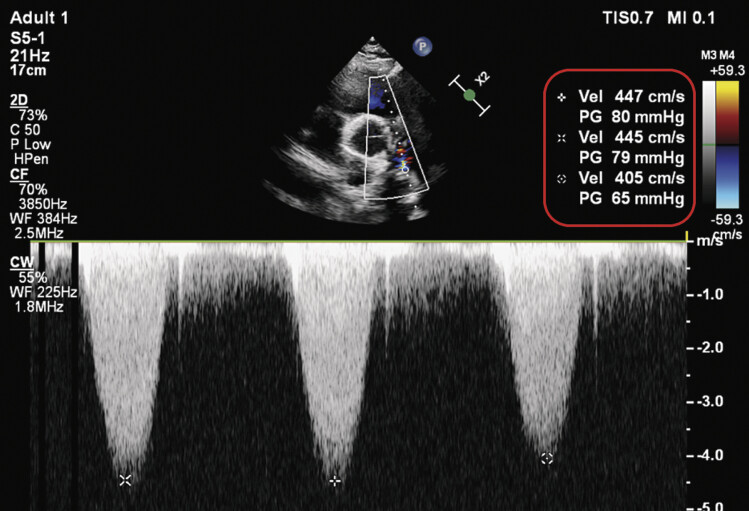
Preoperative echocardiography.
